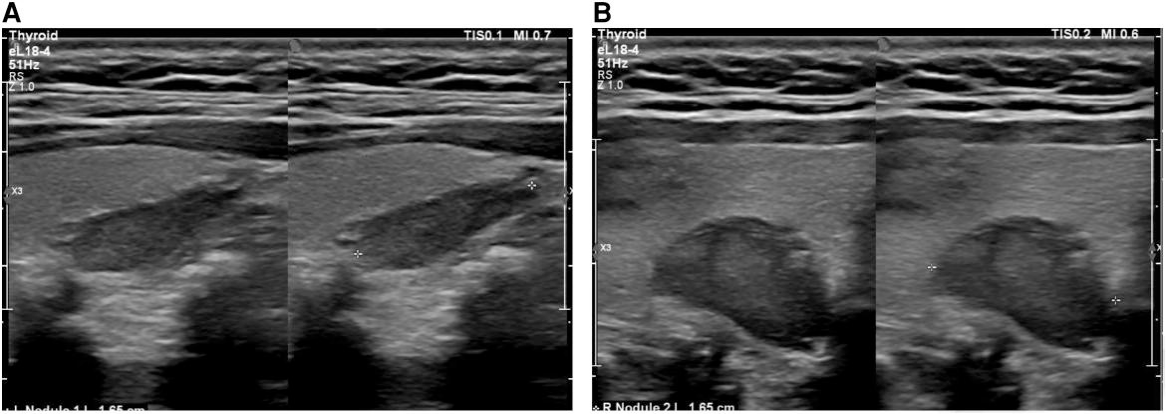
(Case 1): thyroid ultrasound demonstrating bilateral parathyroid adenomas. A, The transverse image of left side of neck showing a well-defined, hypoechoic nodule posterior to the left thyroid lobe, measuring 1.7 cm, suggestive of parathyroid adenoma. B, The transverse image of right side of neck showing a similar hypoechoic nodule posterior to the right thyroid lobe, also measuring approximately 1.7 cm, consistent with parathyroid adenoma.
